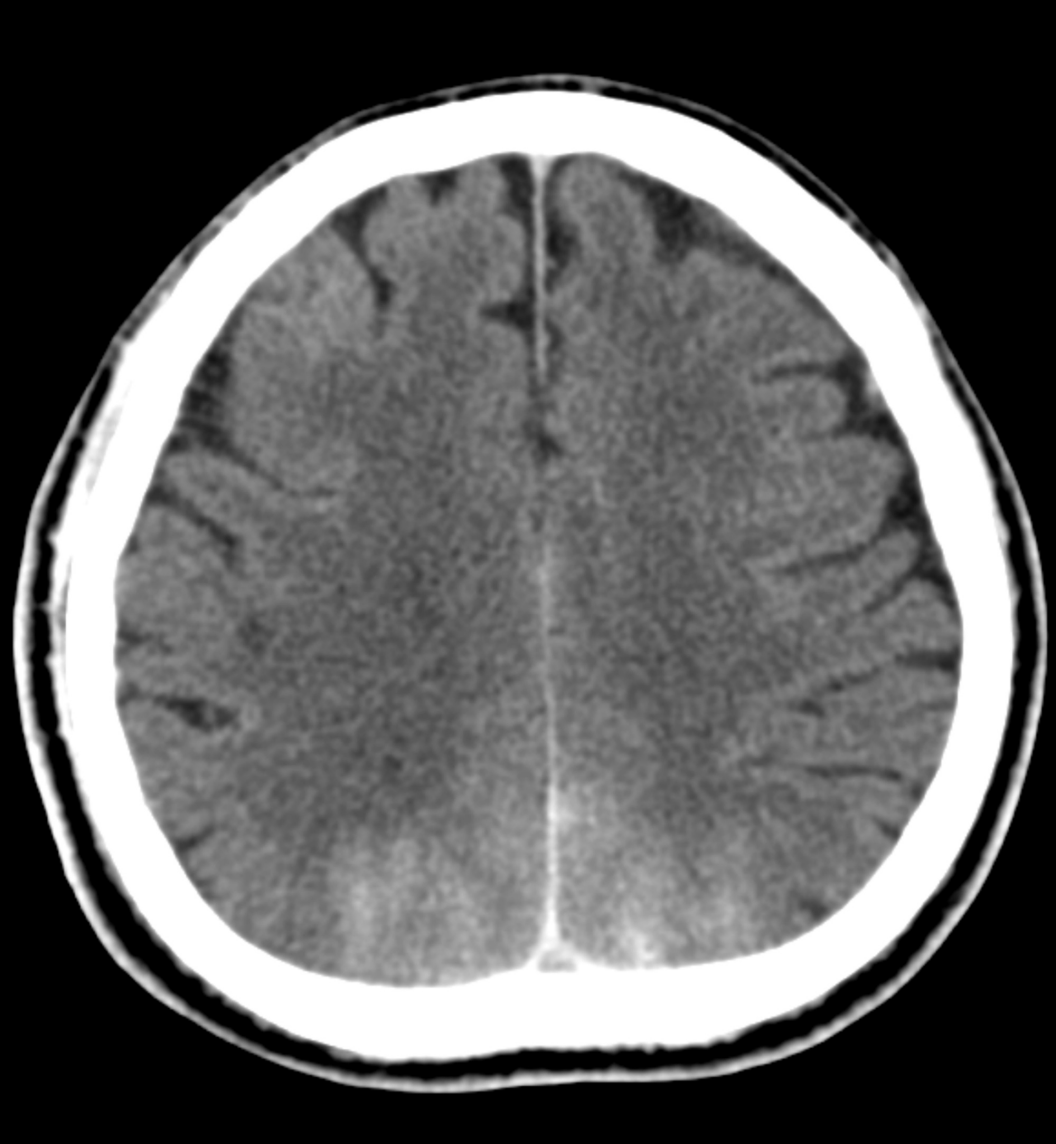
An urgent non-contrasted computed tomography scan of the brain revealed hyperdensities in both occipital lobes, which were reported as acute subarachnoid bleed.
